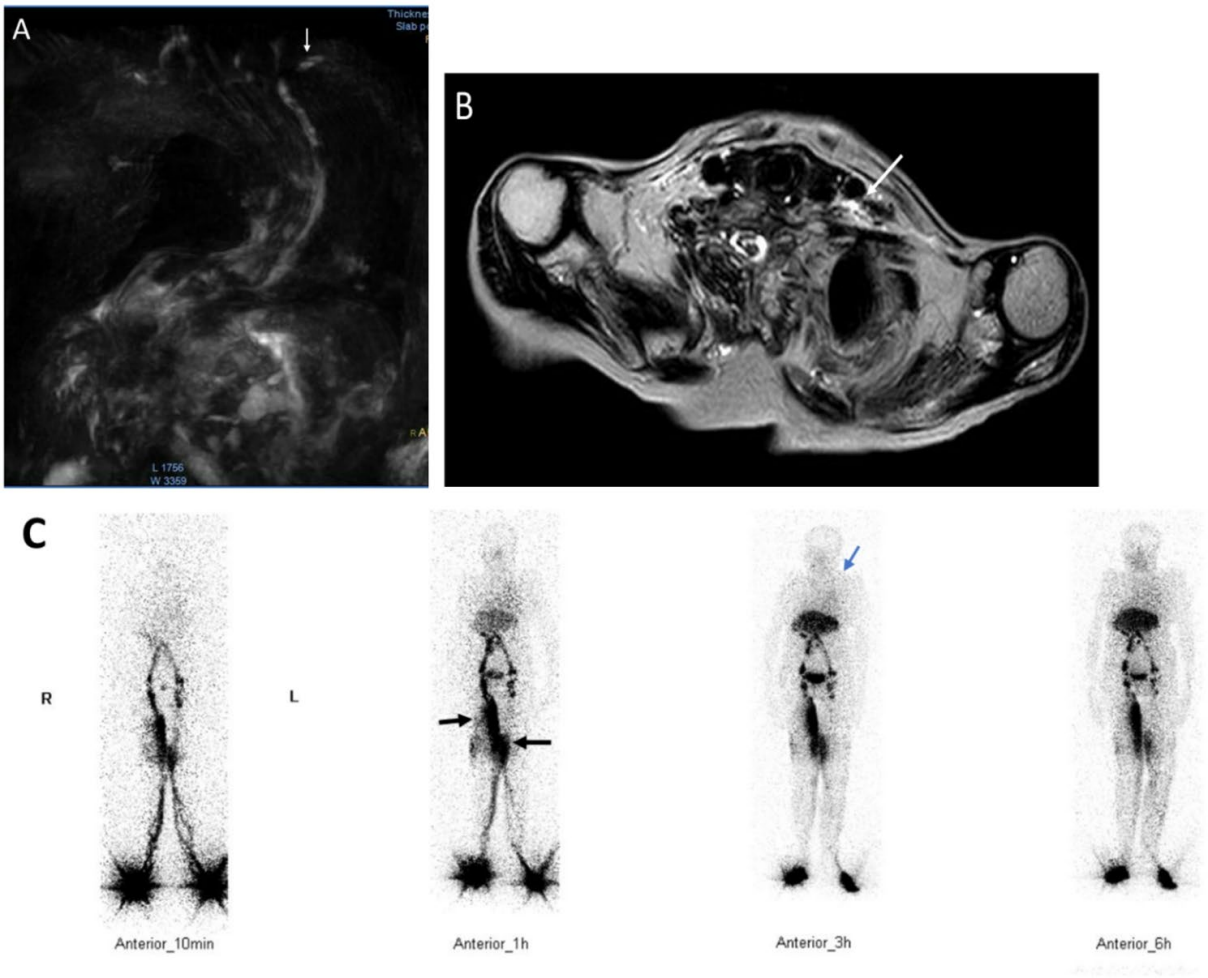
A–C A patient with RA with lymphatic system involvement and scoliosis of the spine. (A) Coronary NCMRL and (B) Axial NCMRL showing no stenosis of the thoracic duct lumen at the left venous angle region, and no abnormally tortuous tubular structures in the surrounding area(white arrow). (C) ^99^TC^m^-DX lymphoscintigraphy (anterior): no contrast agent enhancement at the venous angle (blue arrow), indicating no obstruction at the thoracic duct outle; contrast agent retention in the subcutaneous tissue of bilateral lower limbs (black arrows), suggesting lymphedema
